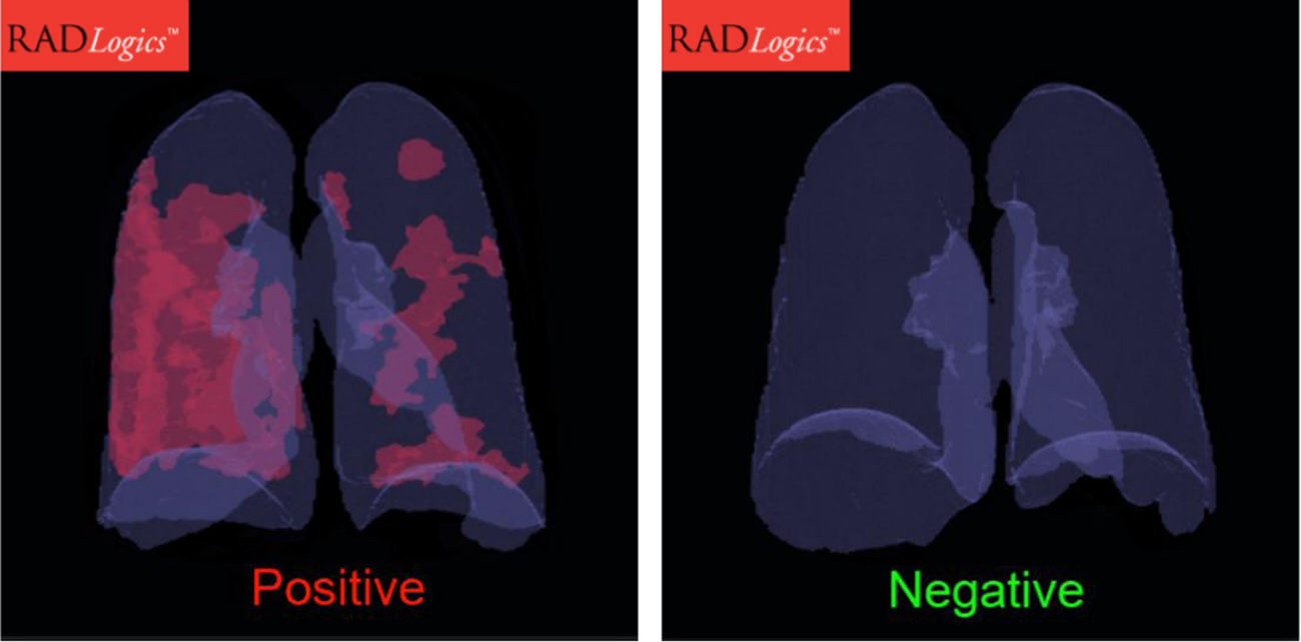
Example of a ML solution for detecting and estimating the extent of COVID-19 infection based on CT images. The image on the left shows a patient infected with COVID-19. The image on the right shows a patient negative for COVID-19. Images courtesy of RADLogics, Inc., used with permission.
